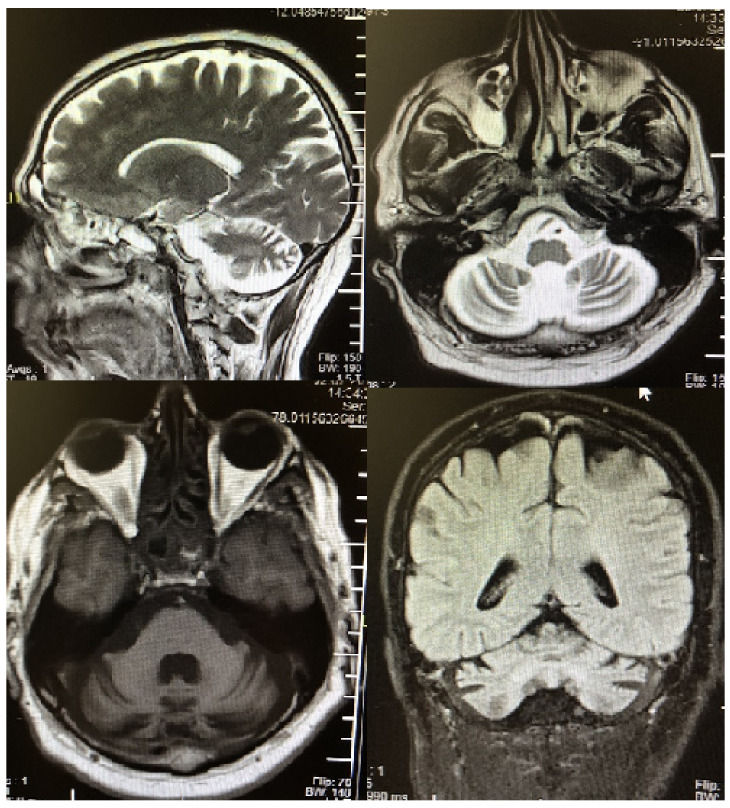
Brain MRI revealed a significant cerebellar atrophy in the superior.
